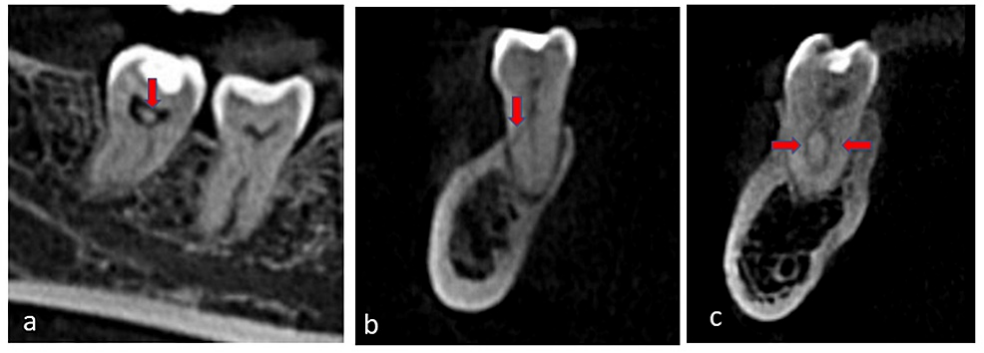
Cone-beam computed tomography images a: Pulp stone in the pulp chamber of the right second mandibular molar, sagittal view (arrow). b: Lateral canal in the distal root of the left second mandibular molar, coronal view (arrow). c: Canal morphology in the mesial root of the right mandibular first molar, coronal view (arrow)
